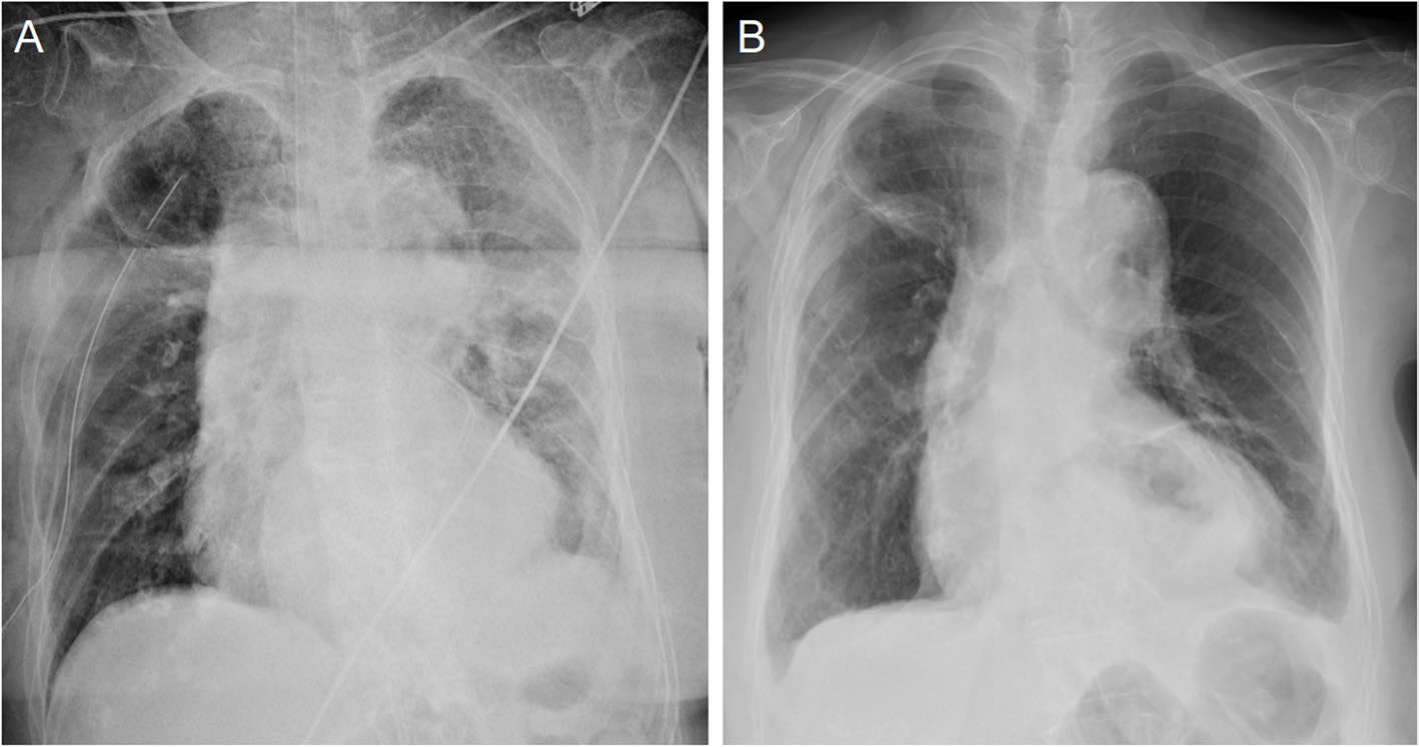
The chest X-ray on postoperative day 1 shows good expansion of the right lung. On postoperative day 9, the X-ray reveals a shadow at the resection site in the right upper lung, but there is no recurrence of pneumothorax
